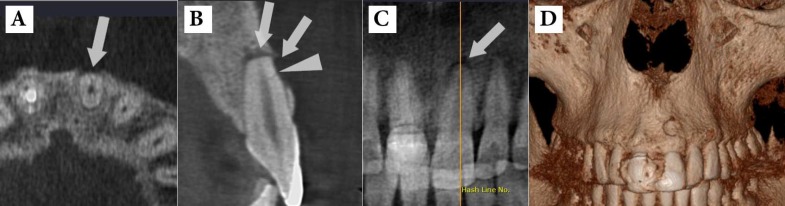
A)* CBCT view shows a tiny horizontal root fracture on the buccal surface of the maxillary left central incisor caused by impact trauma; *B and C) *Note the two separate periradicular lesions in the apical area (arrow) and adjacent to the fracture line (arrow head) due to tooth necrosis; *D*) Three-dimensional reconstruction of the lesion in the periradicular buccal area
